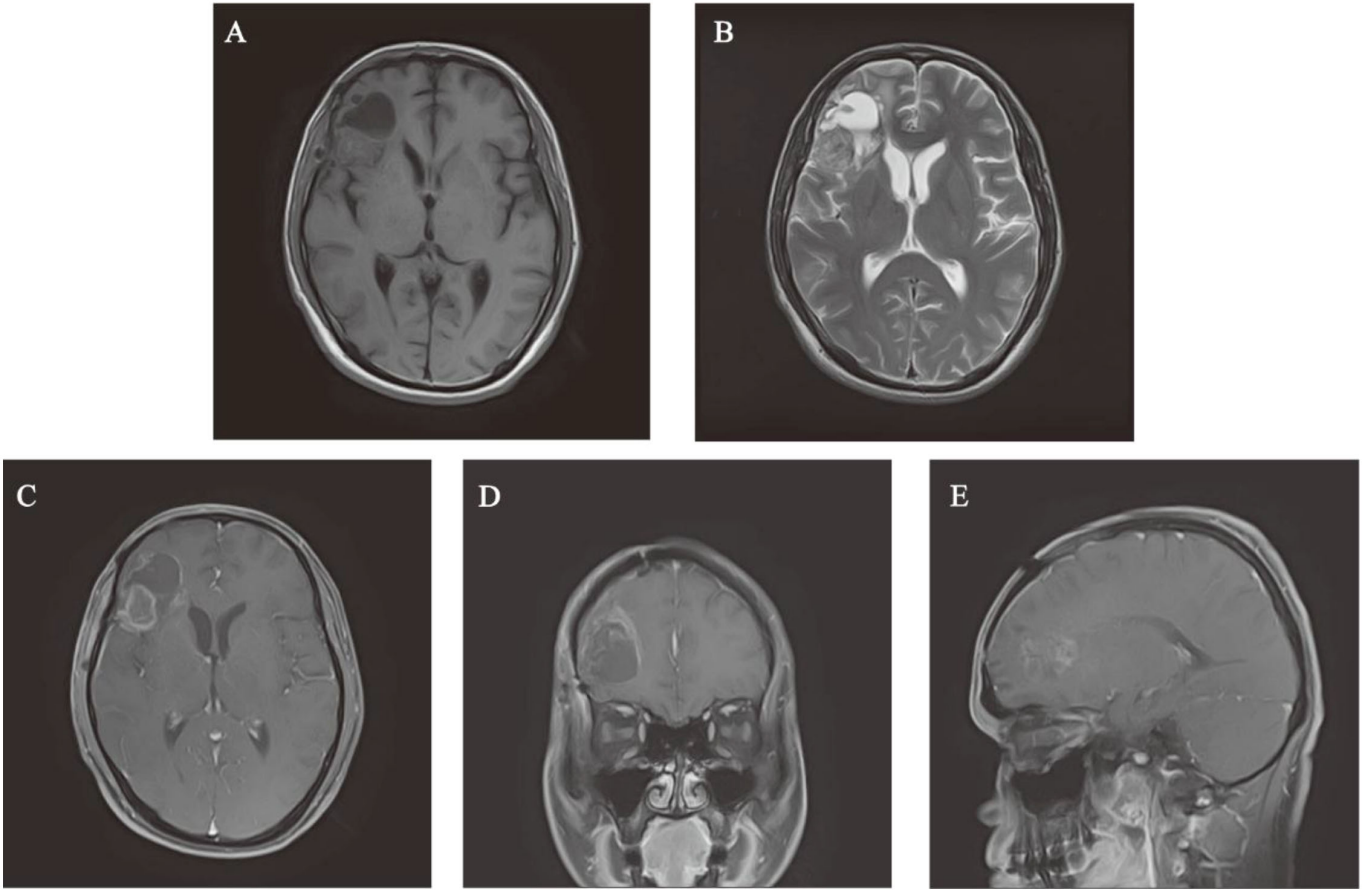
Postoperative MRI images of head. (A, B) T1-weighted sequences and T2-weighted sequences. (C–E) The surgical area lacks notable enhancement on post-contrast T1-weighted MRI, shown in axial, coronal, and parasagittal views.
